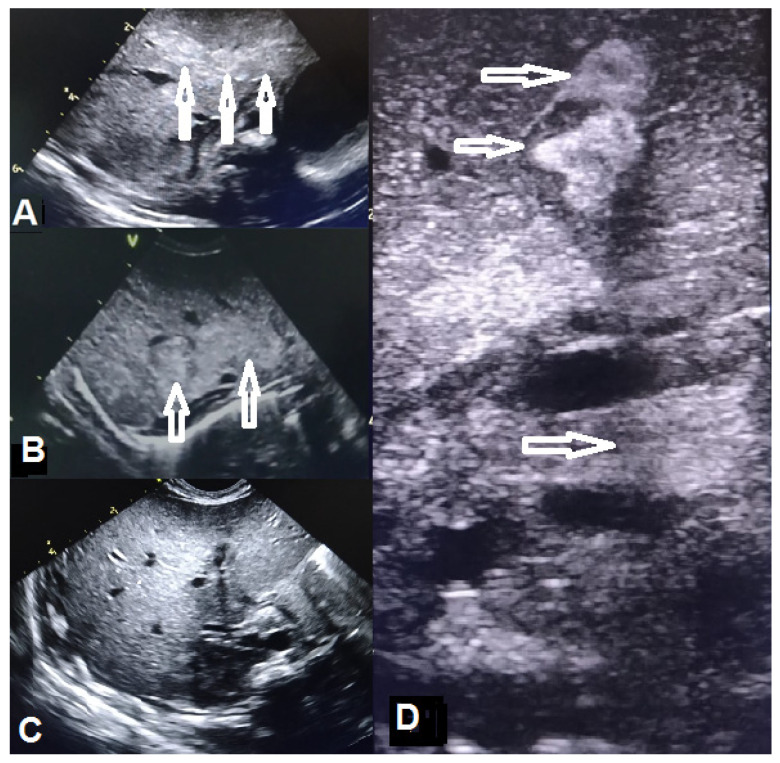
(A) Almost generalized gross, nodular, hyperechoic structure of the liver; (B,D) limited areas of fine granular echogenic hepatic structure; (C) fine, granular, normal sonographic aspect of the liver; arrows are indicating gross, nodular hyperechoic areas.
